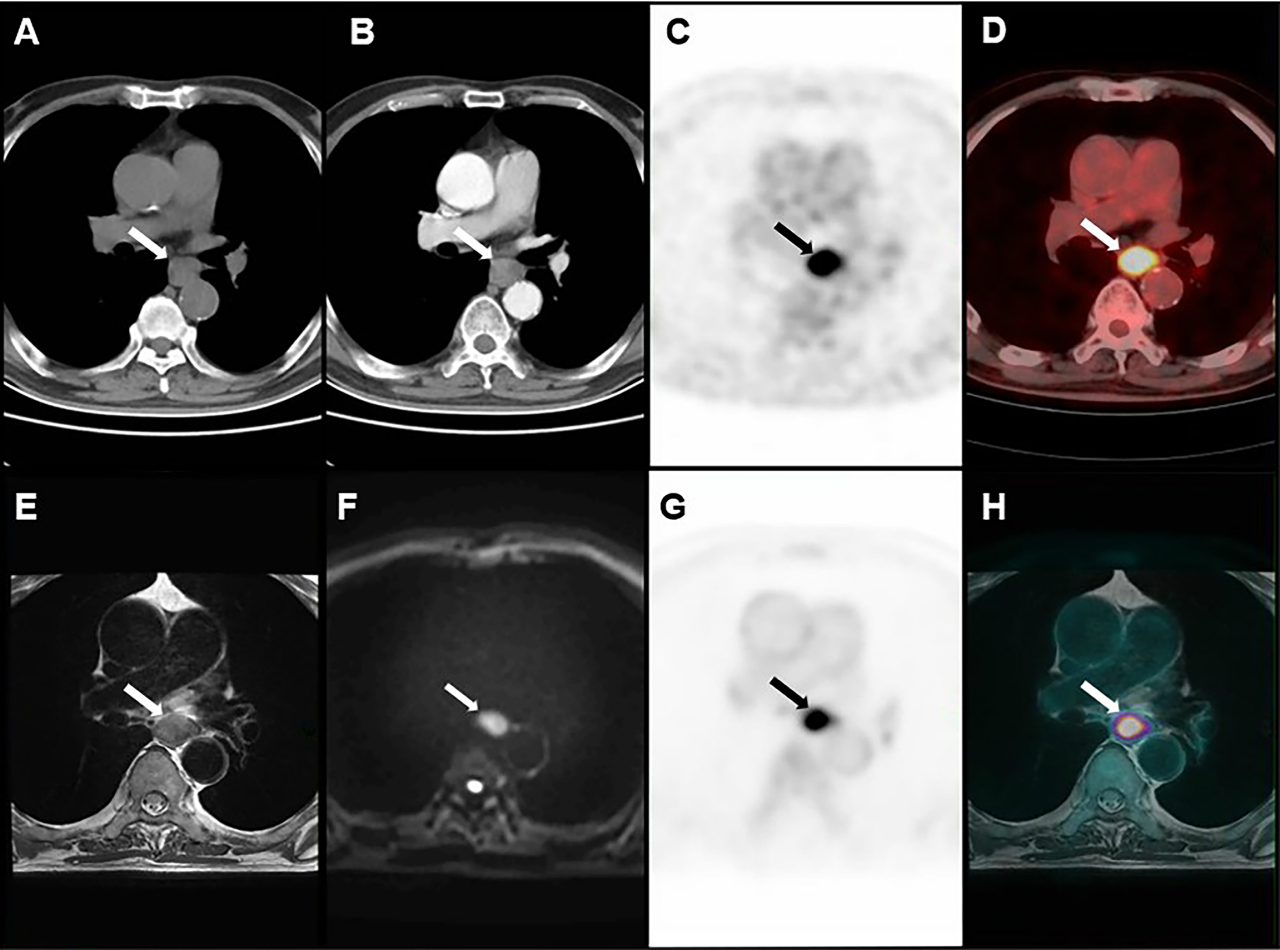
Images of a 72-year-old man with ESCC. (A) plain CT. (B) CECT. (C, D) PET/CT. (E–H) PET/MRI. T3 disease was considered by PET/MRI, which was consistent with postoperative pathology.
